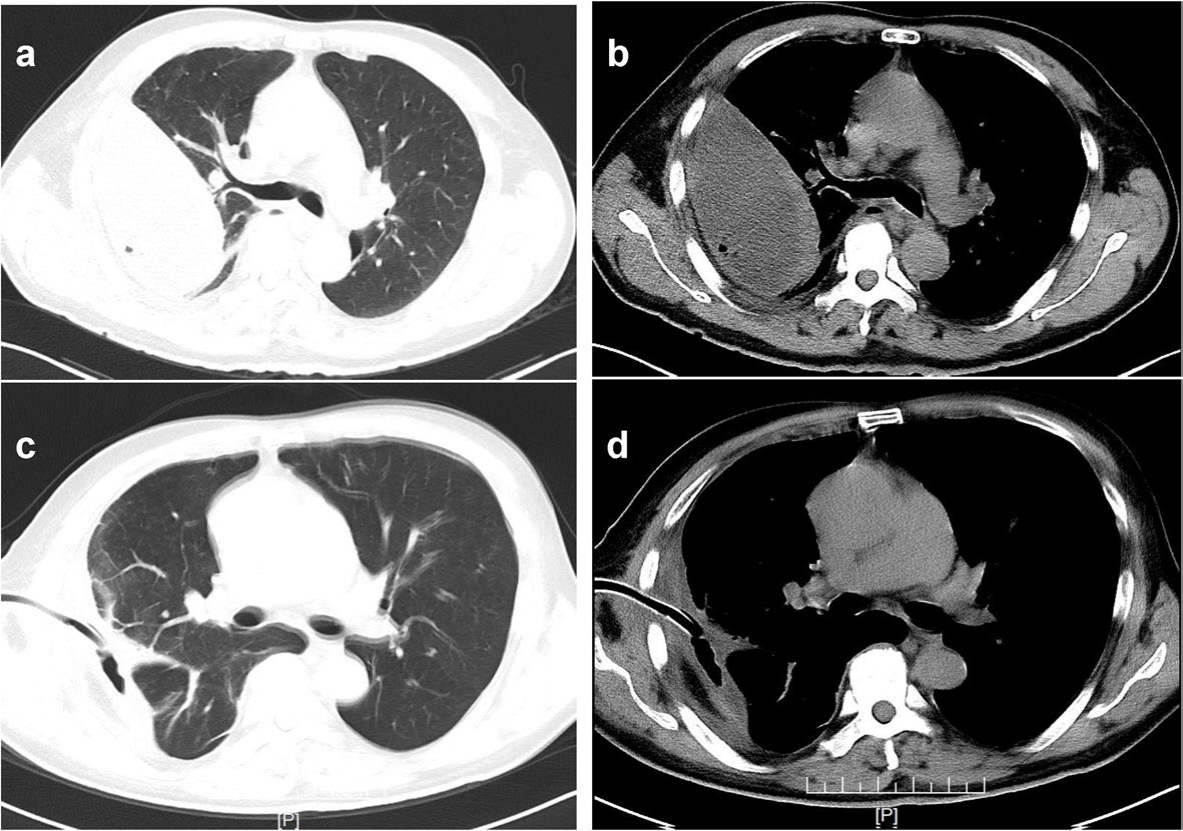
Chest computed tomography (CT) obtained on admission (a) chest window e (b) mediastinal window showing located right pleural effusion. Chest CT performed at two weeks after the start of antimicrobial treatment (c) chest window e (d) mediastinal window demonstrated a decrease in right pleural effusion and thickening of the right pleura
